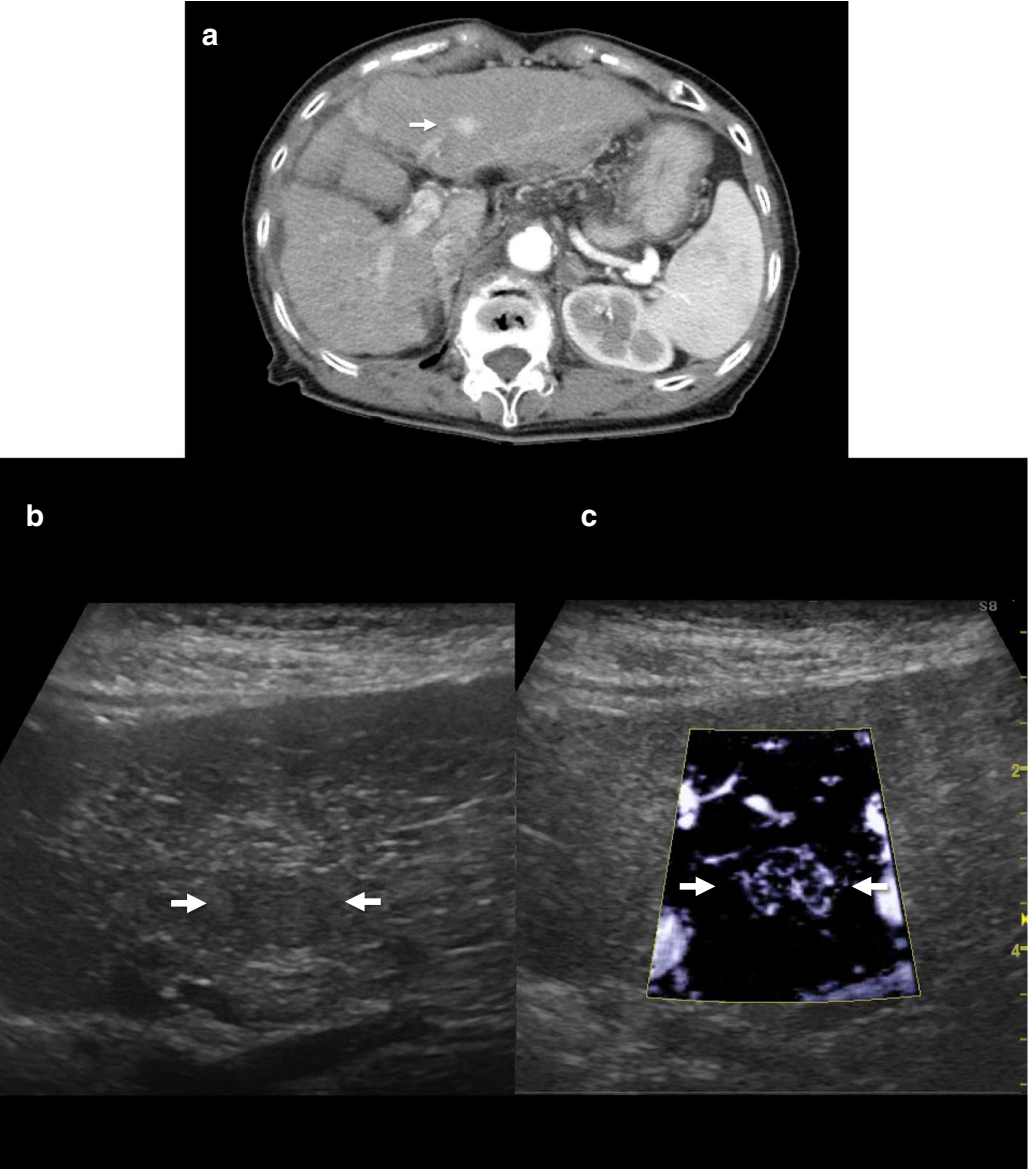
A case of newly developed HCC (maximum diameter 11 mm) in Segment 3. Contrast-enhanced CT in the arterial phase shows a high attenuation area (a). Although the tumor is tiny and hypoechoic (b), high-definition color (HDC) could produce very fine microvascular imaging with high resolution and a high frame rate (c)
